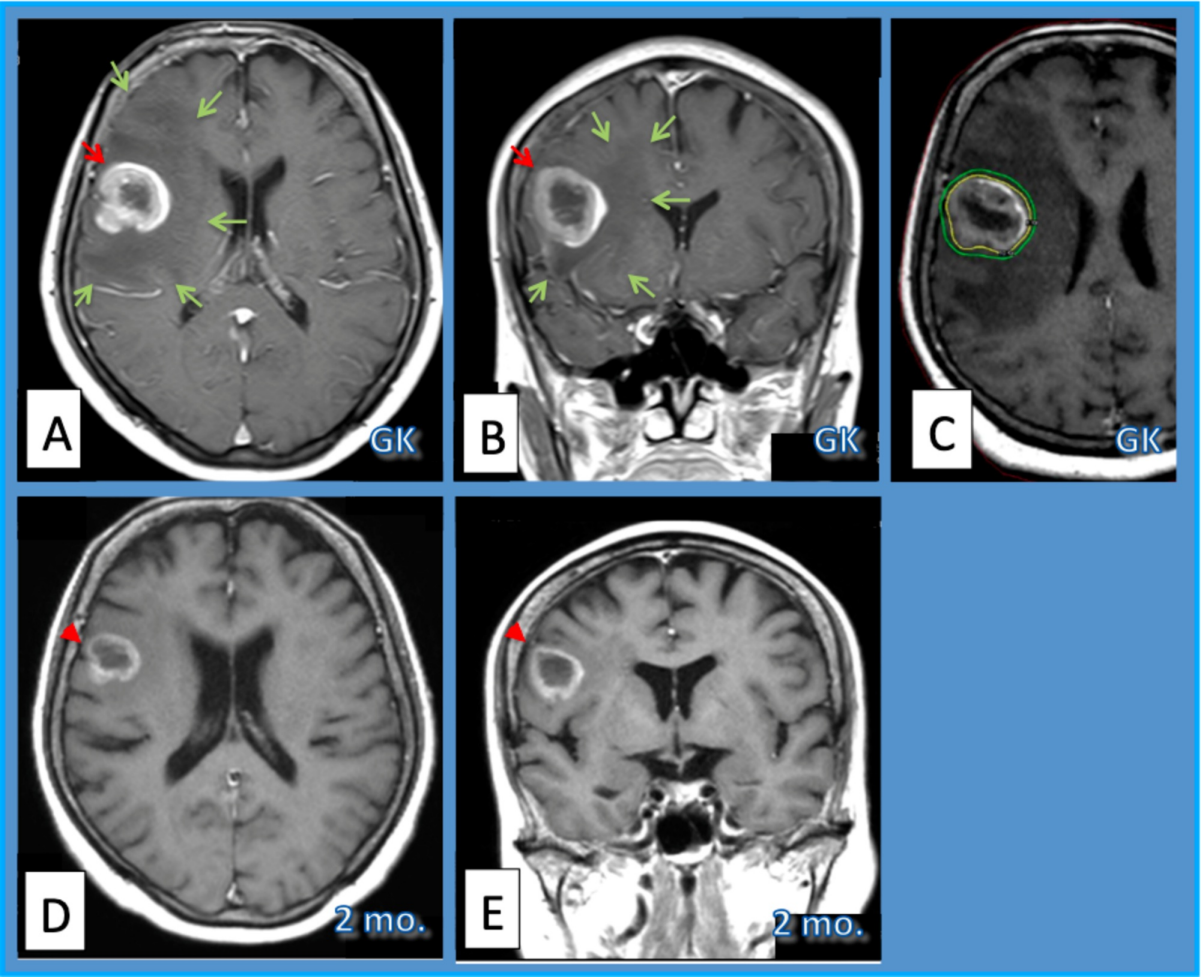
Case 4. Case 4.  Sixty-nine-year-old woman with lung adenocarcinoma brain metastasis. Axial (A) and coronal (B) magnetic resonance images (MRIs) with gadolinium (Gd) enhancement from before Gamma Knife radiosurgery (GKRS) show a right frontal mass lesion (red arrows). A margin dose of 20.4 Gy in 2 fractions (fx.) was delivered for a prescription isodose volume (PIV) of 15.9 ml on GammaPlan (Elekta, Tokyo) workstation (C). The yellow line shows prescription isodose and the green line indicates the isodose line of 16 Gy/ 2 fx. The lesion shrank somewhat (red arrowheads) and the peritumoral edematous area of low-intensity (green arrows) became less wide within two months after one-day two fraction GKRS (D: axial, E: coronal MRI).
